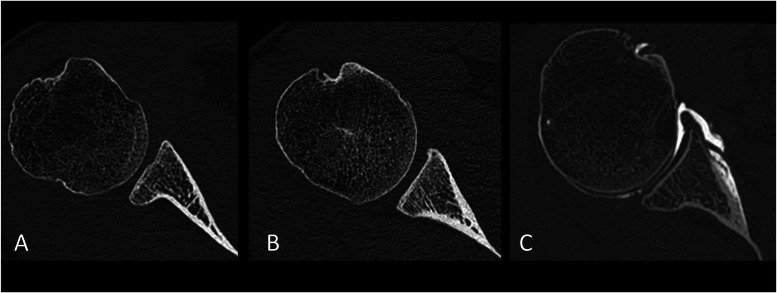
Subjective evaluation of the glenoid articular surface concavity on axial CT images at the mid-level of the glenoid. A) Convex glenoid articular surface in the right shoulder of a 21-year-old female with clinical hyperlaxity. B) Flat glenoid articular surface in the right shoulder of a 28-year-old male with clinical shoulder hyperlaxity. C) Concave glenoid articular surface in the right shoulder of a 54-year-old female with no signs of shoulder hyperlaxity.
